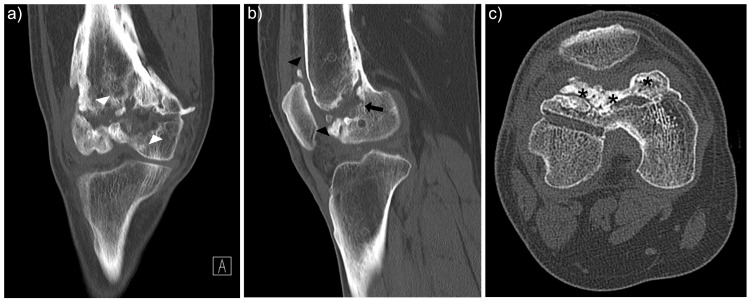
Pre-operative computed tomography (CT) images of the right knee (a) Coronal view demonstrating irregularity and bone loss (white arrowhead) over the anterior distal femur
